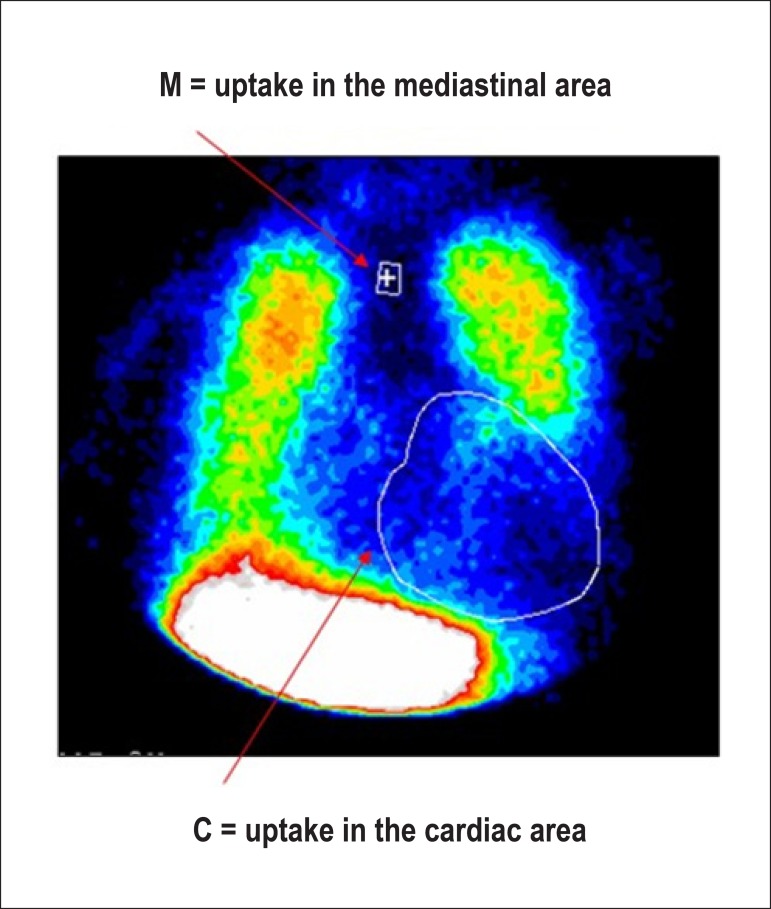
Cardiac scintigraphy with MIBG-^123^I. Colored late imaging of the anterior thorax with the regions of interest (ROI) drawn in the mediastinal (M) and cardiac (C) areas, to calculate the heart to mediastinum ratio (HMR) of MIBG-^123^I uptake. This index is the measure of the ratio between the statistical count of the rate of average radiation per pixel in the ROI, drawn in the heart and mediastinum.
